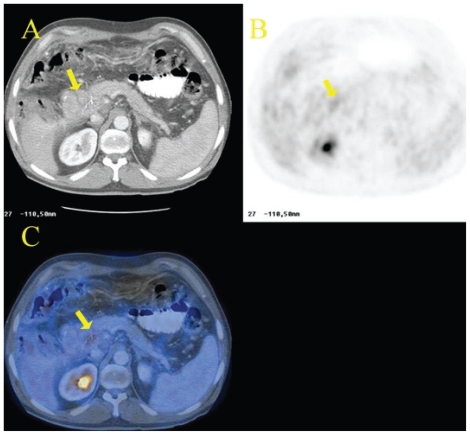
Recurrence of cholangiocarcinoma in a patient who had undergone surgical tumor resection. The CT scan (A) shows a mass at the site of previous surgery in contact with the pancreatic head (arrow). The lesions shows minimal ^18^FDG uptake on ^18^FDG PET (B, D) and ^18^FDG PET/CT fusion (C) images (arrow).
